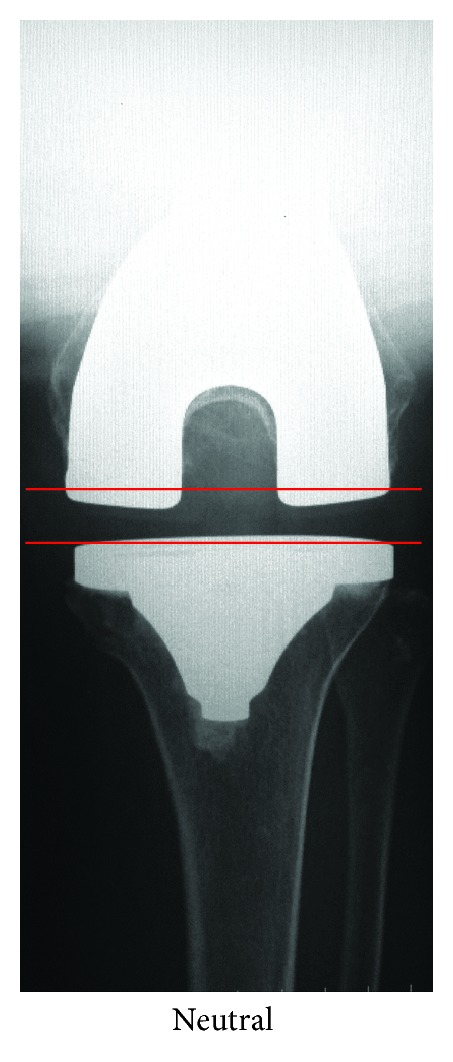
Postoperative axial radiographs of the left distal femur. Patients sit on a table with their lower legs dependent and a 1.5 kg weight attached to the ankle on the treated side. The angle between the cut lines of the posterior femoral condyles and the proximal tibia represents the flexion gap balance (FGB).
